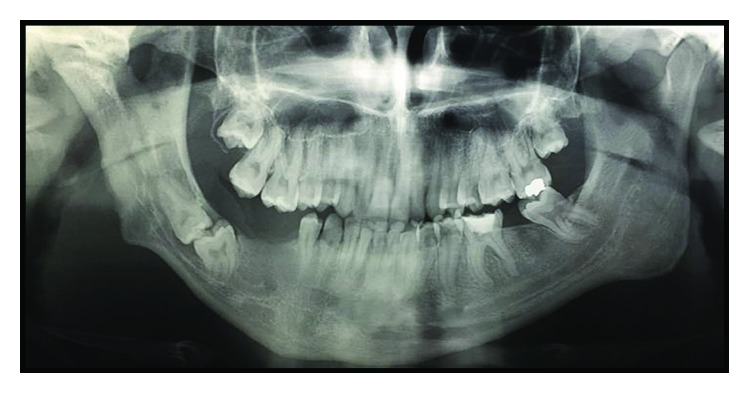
Preoperative panoramic radiography showing altered condylar morphology on the right side, mandibular asymmetry, and impaction of the teeth 47 and 48.
